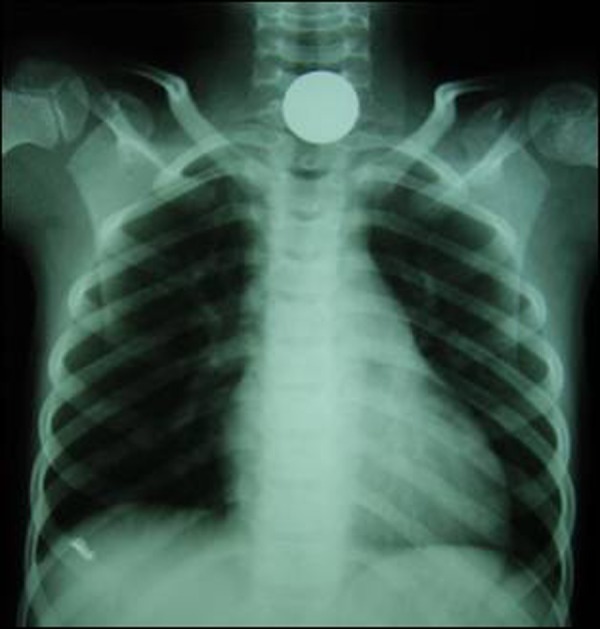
Figure 2: X-ray neck and chest: Showing opaque shadow of a 5 Rupee coin in the cervical esophagus in the 1st patient
